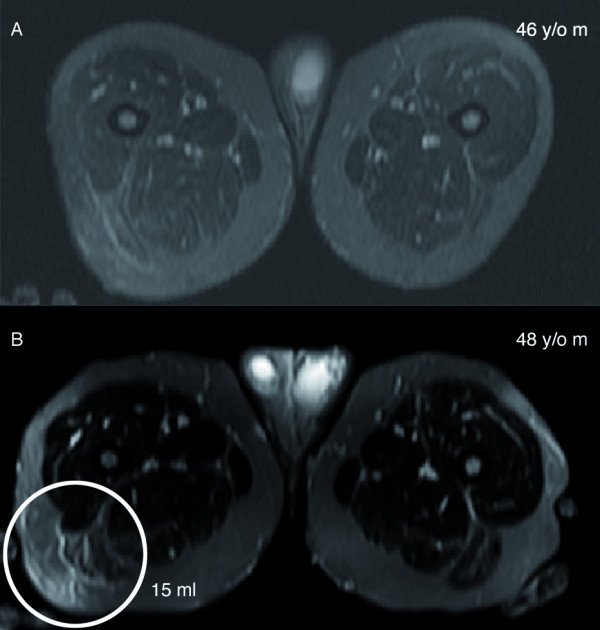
Development of a new PN on MRI; the only PN that arose during the period of observation in this study is shown in this figure (Patient 1670, Tumour 5). A: cross-sectional image through the thigh at age 46 years; B: cross-sectional image through the thigh at age 48 years.
